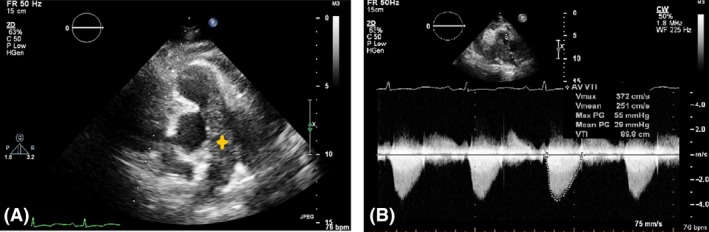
Panel (A) Parasternal short‐axis view of major arteries in TTE. A large, lobulated intravascular mass extending from the vicinity of the PV into the RPA with partial occlusion of the lumen is showed. Panel (B) Continuous‐wave CW Doppler of the pulmonary artery. A significant peak pressure gradient (PPG: 55 mmHg), suggestive of partial occlusion of the main PA is showed. PA, pulmonary artery; RPA, right pulmonary artery; PV, pulmonary valve.
